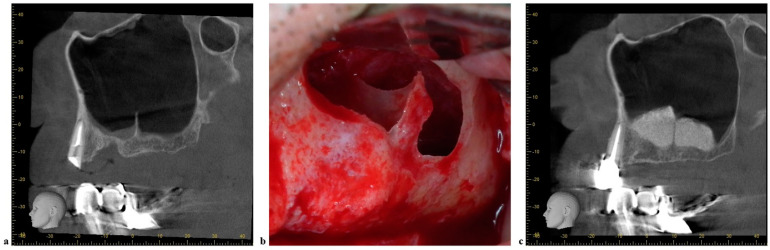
Management of medio−lateral septa. (a) CBCT sagittal view of the left maxilla with a medio−lateral oriented septum. (b) One window with a modified design of the osteotomy. (c) CBCT sagittal view after maxillary sinus floor augmentation.
